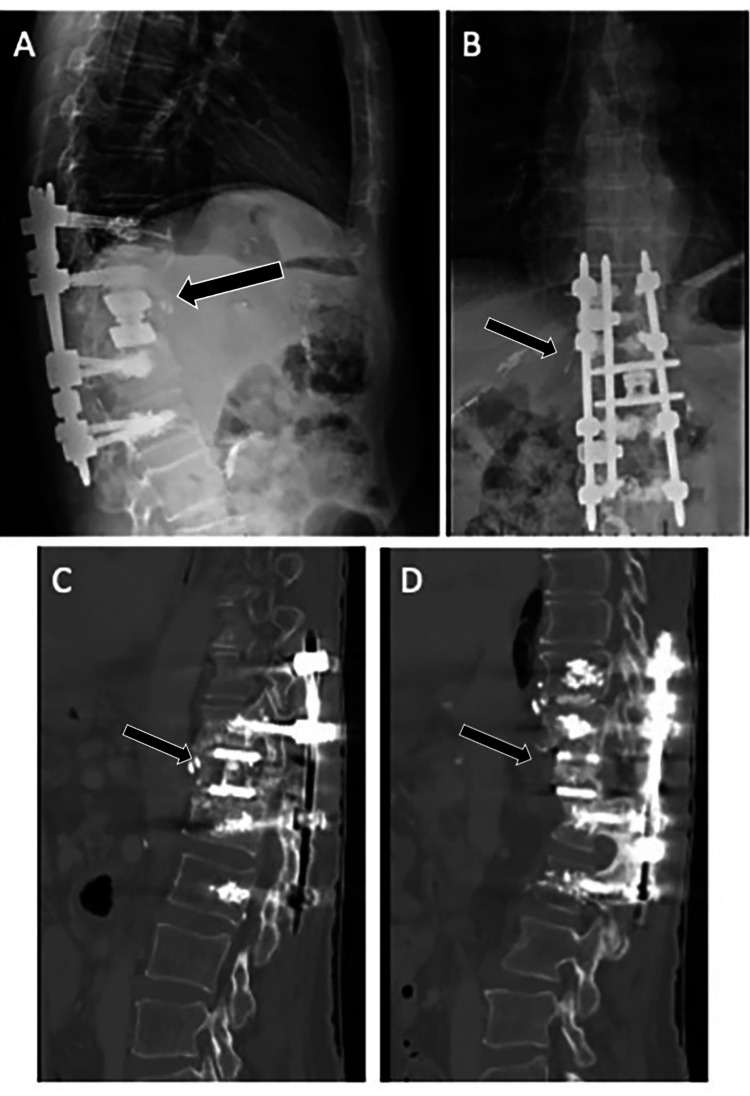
Postoperative imaging. Postoperative radiographs (A) and (B) at three months and postoperative CT (C) and (D) at one year demonstrating stable hardware and arthrodesis across revision construct. CT: computed tomography.
